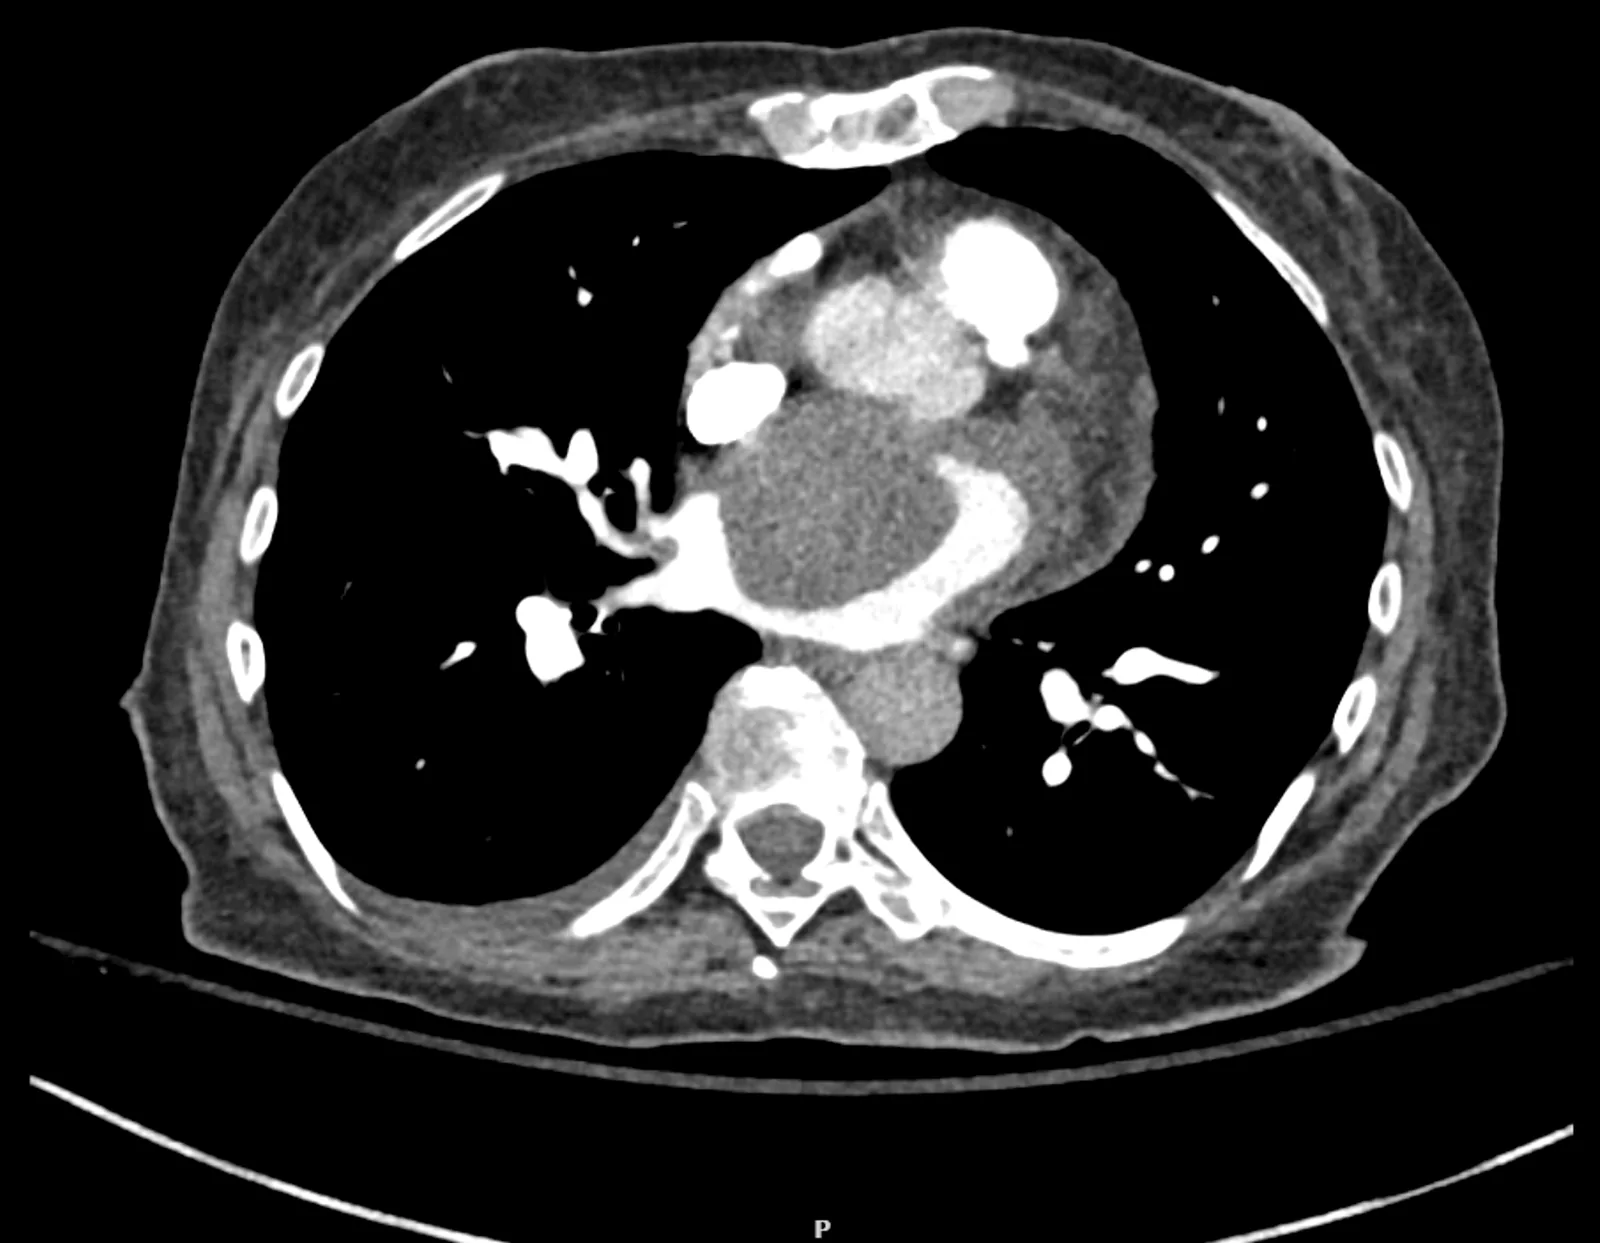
A repeat CT angiography chest. Repeat CT angiography demonstrates progression of the left atrial mass. Axial contrast-enhanced image demonstrates a large, heterogeneous hypoattenuating mass measuring approximately 5.2 cm in maximal dimension, occupying most of the left atrial cavity. The mass is lobulated and has a broad interface with the atrial wall, with marked narrowing of the residual contrast-opacified atrial lumen. These findings, together with the substantial interval enlargement from earlier imaging, favor an infiltrative cardiac neoplasm over organized thrombus.
